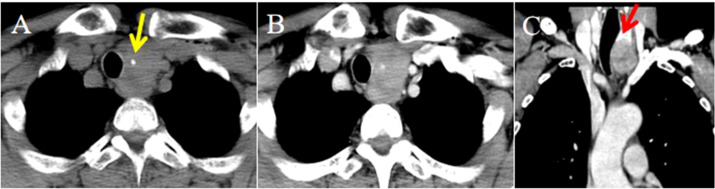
CT imaging of the ITTC. (A) Plain CT scan showed an irregular mass at the left tracheoesophageal groove with extension to the thyroid’s lower pole, with central calcification (yellow arrow); (B) After the injection of contrast medium, it exhibited slight to mild heterogeneous enhancement with small central hypodense areas; (C) Compared with uninvolved thyroid tissue, the tumor-thyroid interface forming an arc-shaped boundary at multiplanar reconstruction (red arrow).
